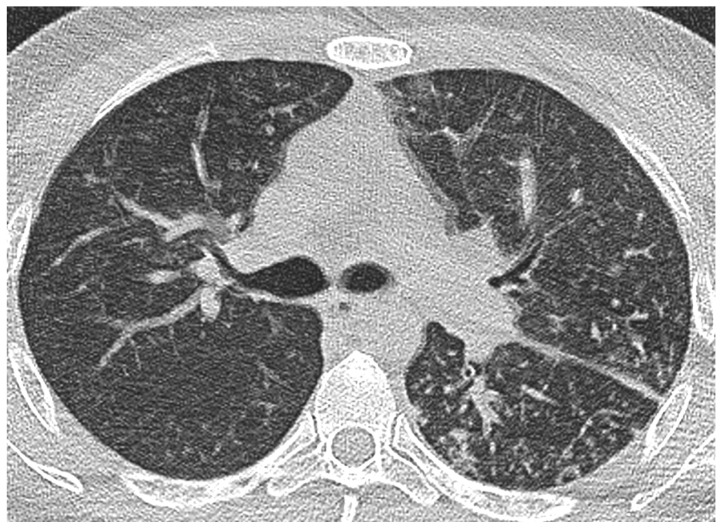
Chest CT: unilateral interstitial infiltrates, appearing as diffuse reticular basal bronchopneumonic opacities, consistent with atypical pneumonia due to *Mycoplasma pneumoniae*. Representative image from the anonymized institutional radiology archive of University Hospital Frankfurt.
